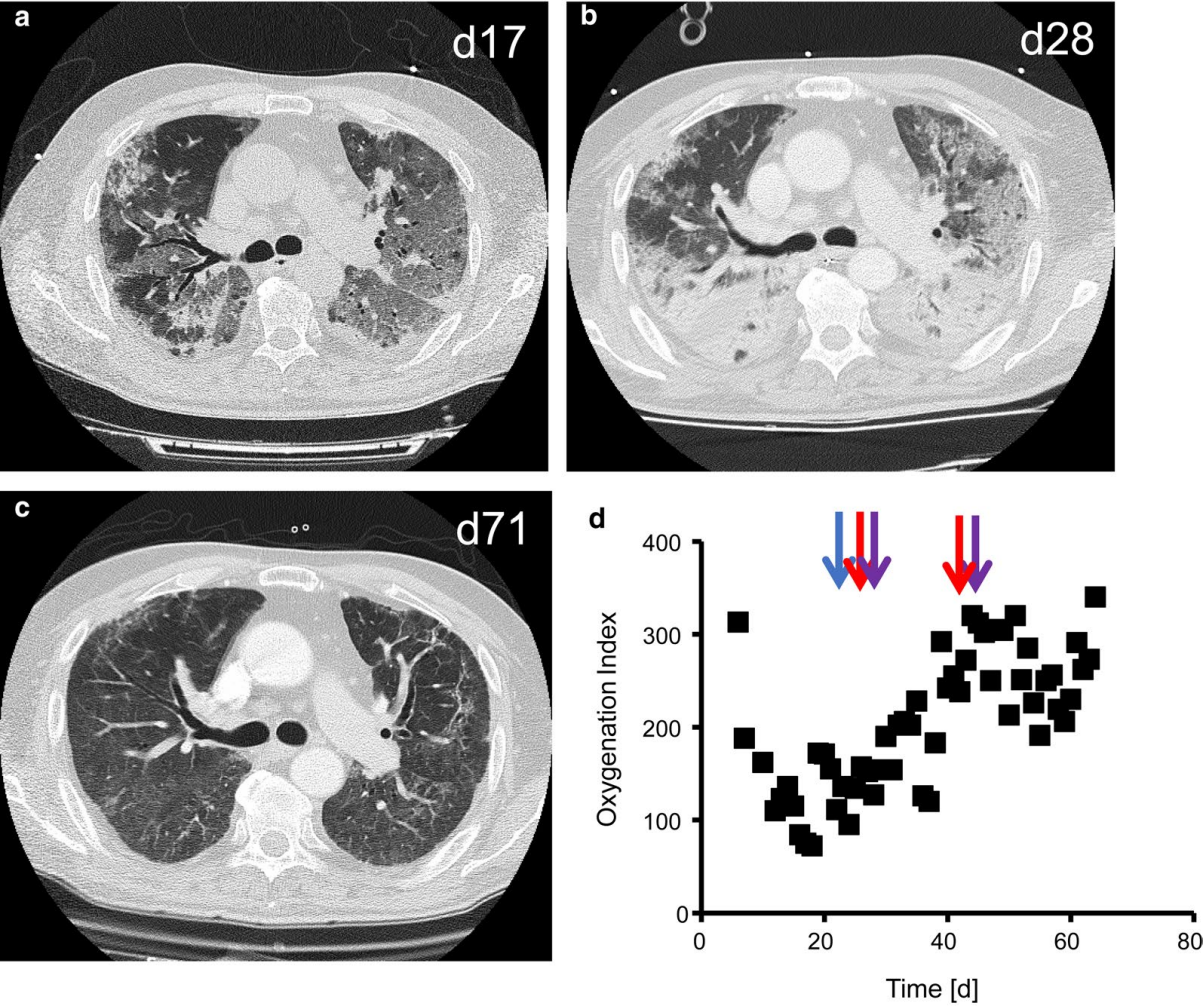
Patient 2. Chest computed tomography showing progressive interstitial pneumonia on day 17 (a) and day 28 (b) with dense and partly consolidated infiltrates, fine reticular pattern and no honeycombing. Dramatic improvement in the chest computed tomography scan on day 71 (c). There was a close temporal association of the initiation of therapeutic plasma exchange on day 24 (blue arrow), and the infusion of cyclophosphamide (red arrow) and rituximab (violet arrow) on day 28 and 42 with recovery of oxygenation index (closed squares) (d)
